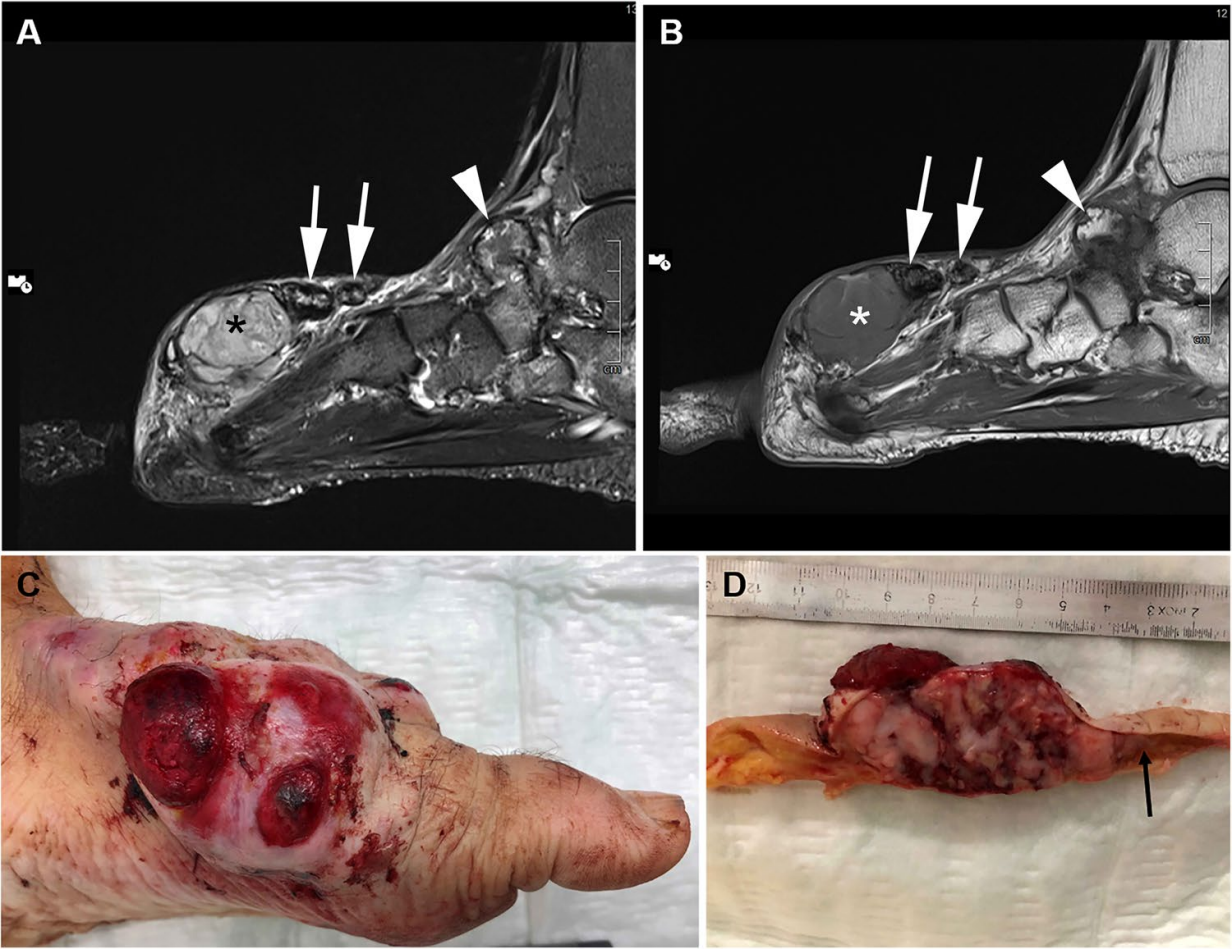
Representative examples of the imaging, clinical and gross findings. Sagittal fat suppressed (fs) T2-weighted MRI (A) shows a subcutaneous multi-lobulated mass of the midfoot dorsum. The lesion is well-demarcated by a hypointense rim and of heterogeneous high signal intensity (black asterisk). In addition there are smaller oval-shaped lesions continuous with the main mass exhibiting a hypointense rim in T1 and T2 fs reflecting hemosiderin deposits (arrows in A and B). The corresponding T1 weighted image shows heterogeneous hypointensity of main lesion (asterisk in B). A large osteophyte or osteoproliferation due to severe talonavicular osteoarthritis not related to the mass is highlighted by arrowheads in A and B. C Extensively ulcerated large multinodular mass bulging from the dorsum and medial aspect of the foot. D Cut-surface shows soft fleshy tan to reddish mass with extensive areas of necrosis and hemorrhage. Note brownish tissue on the right corresponding to the HFLT component (arrow)
